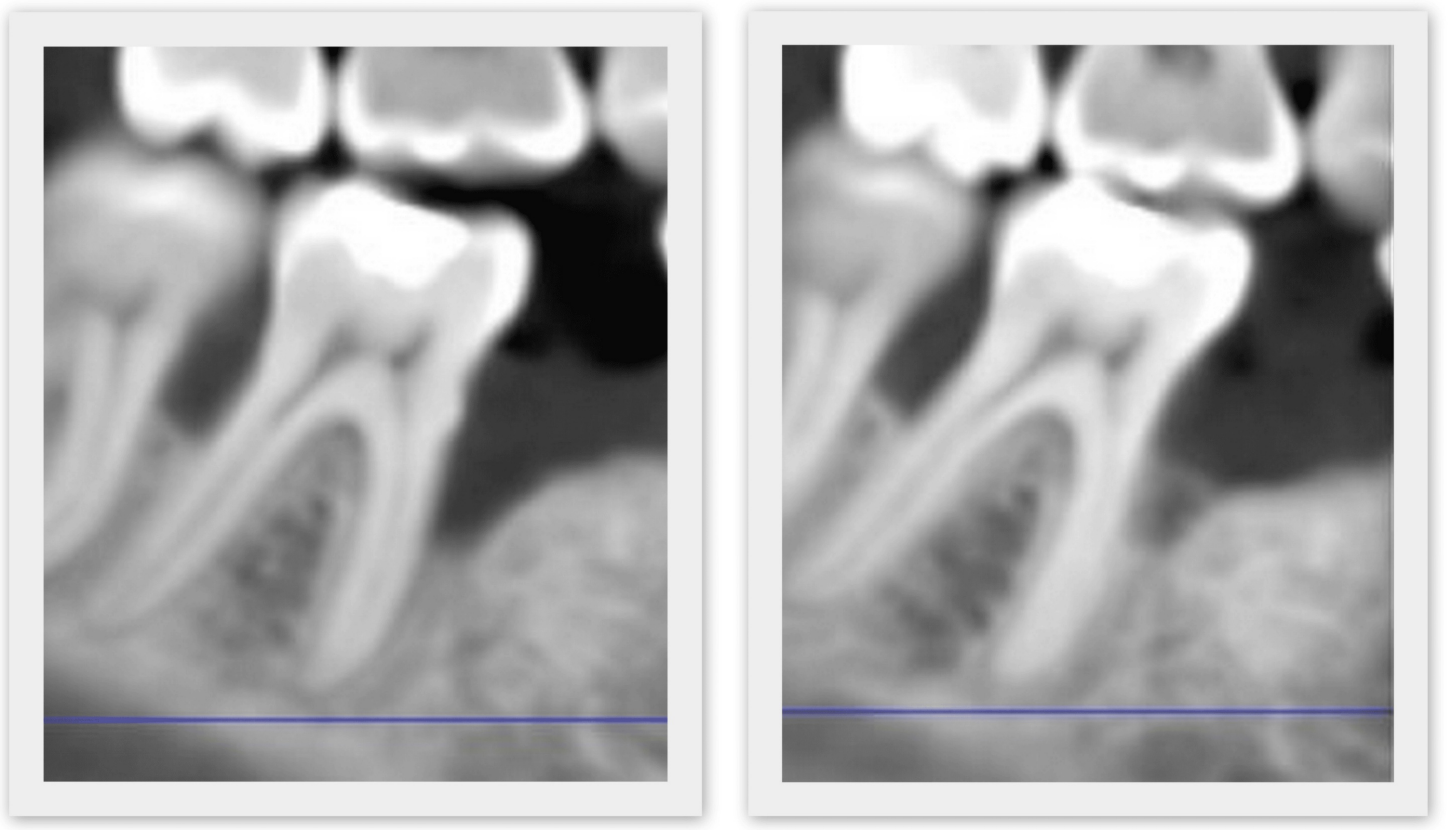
Cone beam computed tomography imaging before and after regenerative therapy.
